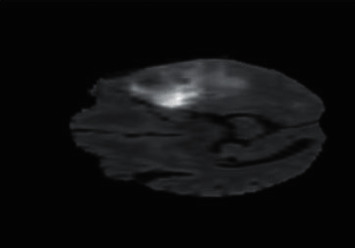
Image of FLAIR (BraTS17_13_2_1).
